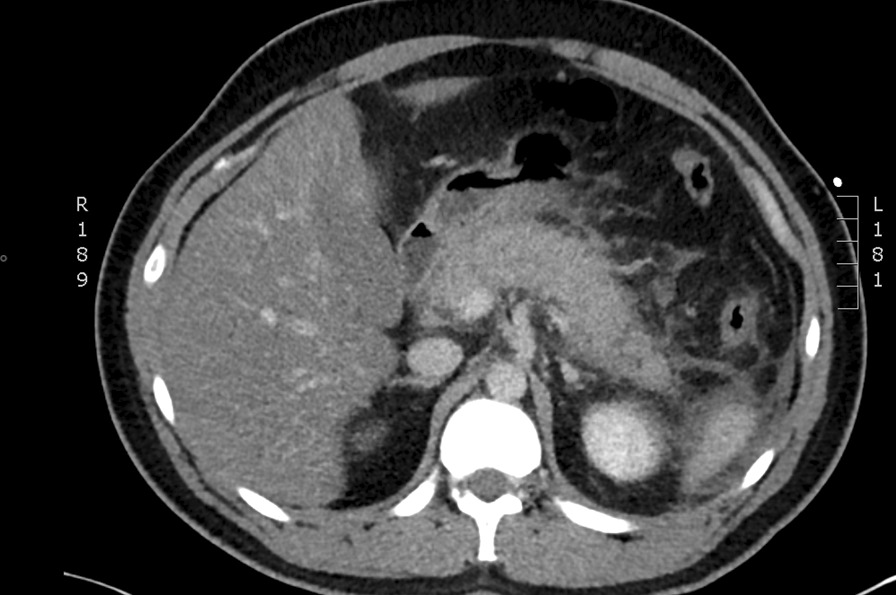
Abdominal computed tomography. Pancreatic oedema with surrounding fat stranding
